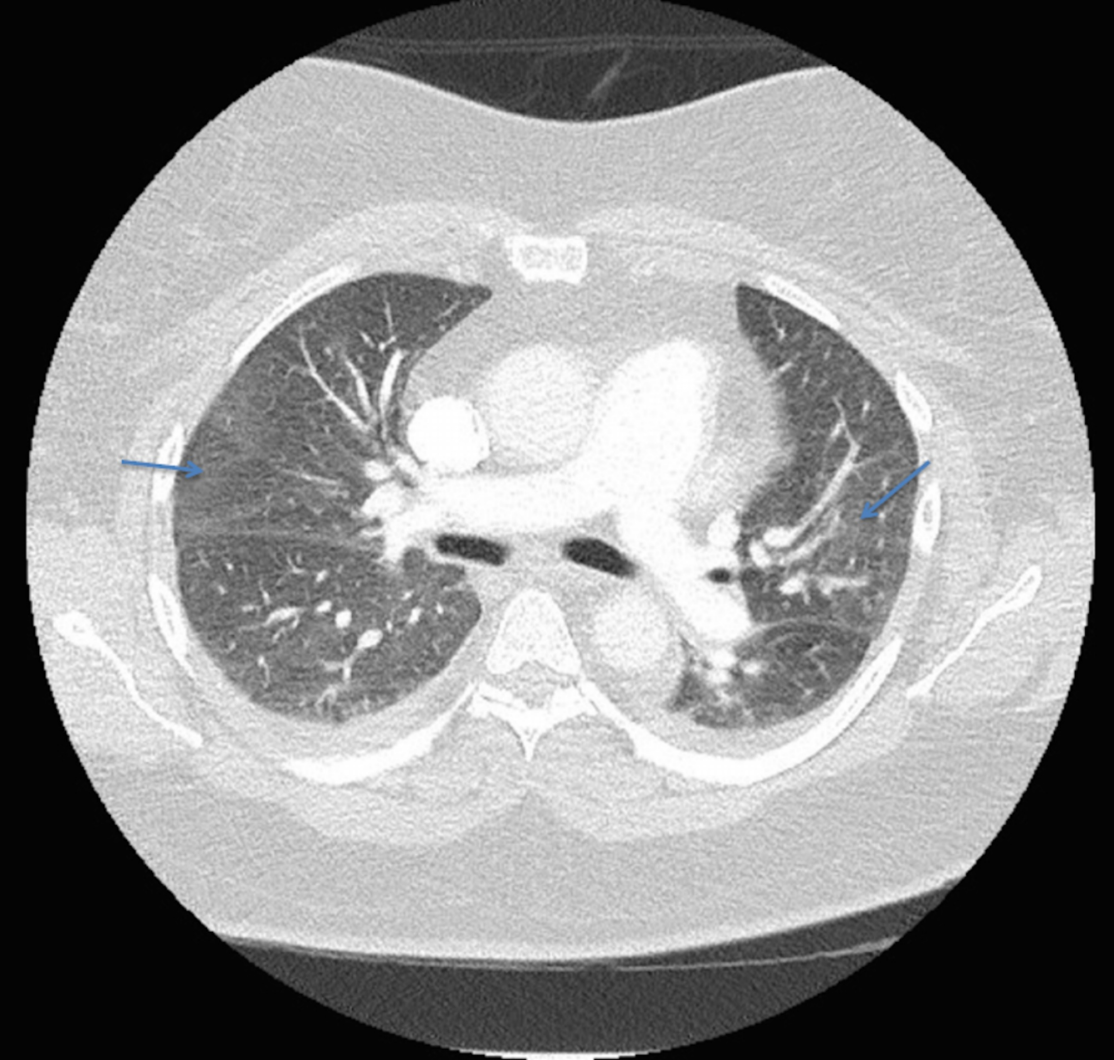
Computed tomography of chest with contrast Computed tomographic imaging of the chest revealed no pulmonary embolism, but multifocal ground-glass opacities were observed (arrows).
